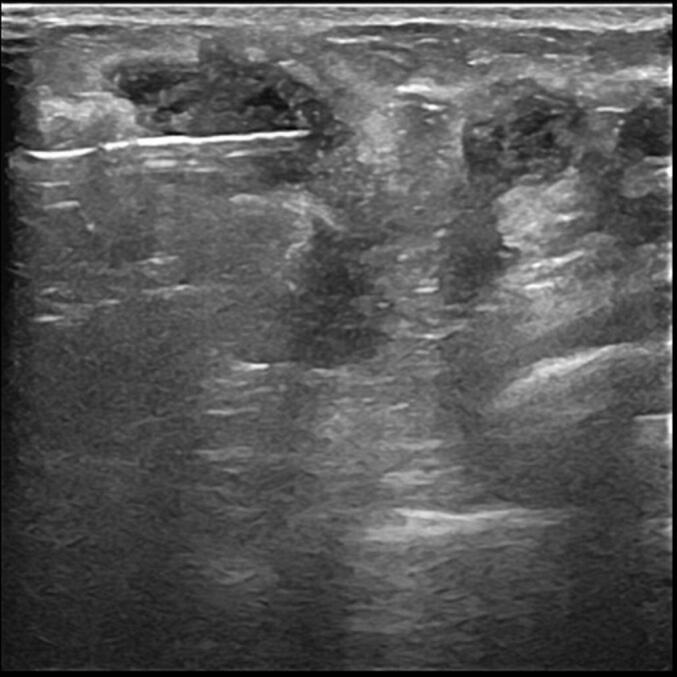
The ultrasound findings showed a hypoechoic formation with well defined and lobulated contours, wich was biopsied.
